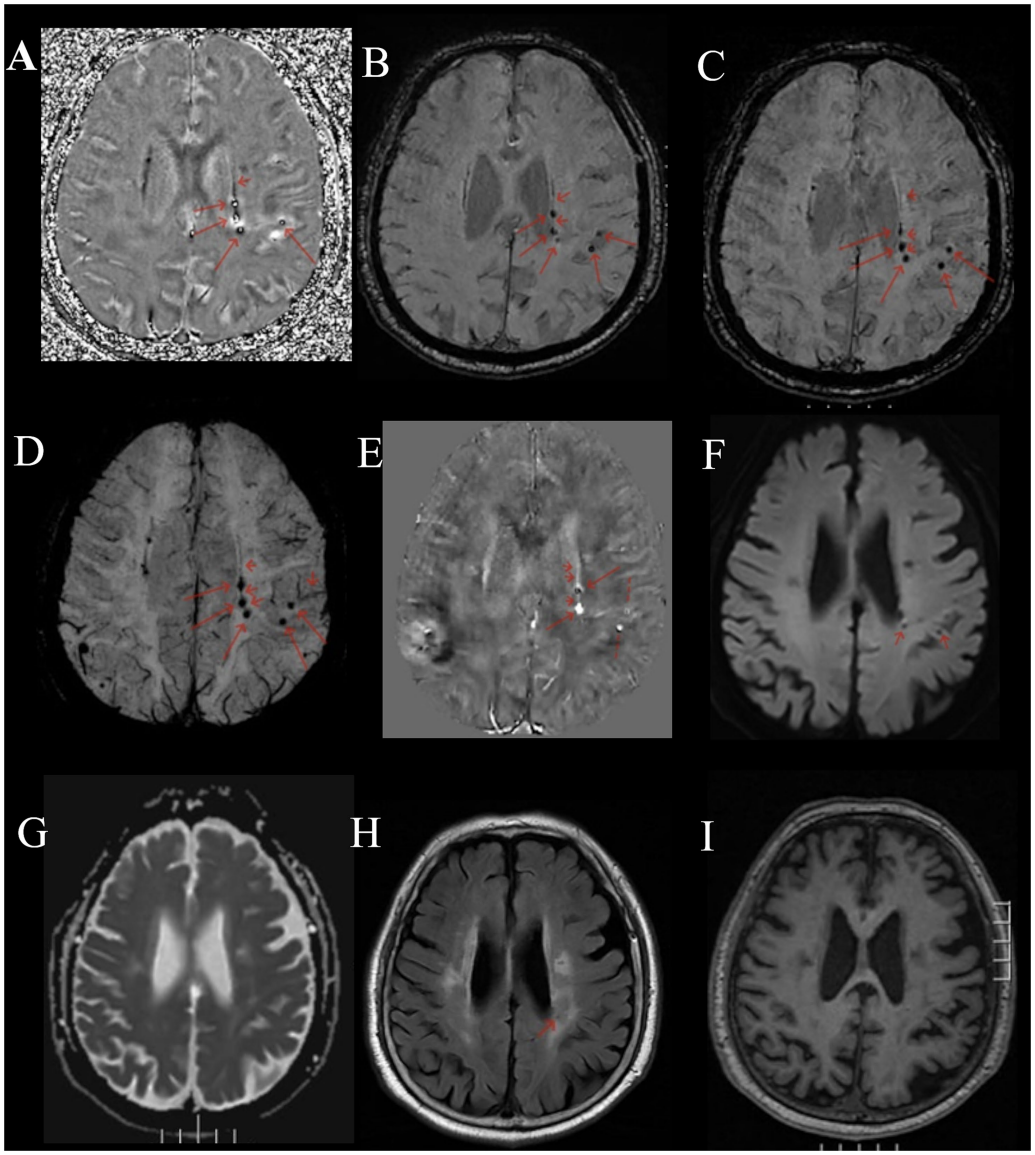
Male, 73 years old, with right limb motor impairment for 15 years and dizziness for 1 day. (A) Phase map. (B) Amplitude map. (C) SWI image. (D) MinIP image with TE of 22.5 ms. (E) QSM image. (F) DWI image. (G) ADC map. (H) FLAIR image. (I) T1WI image. (A–D) Round-like hypointense signals can be seen in the left periventricular region and the left parietal cortex, with hyperintense signals in the center, forming a typical “target sign” (long red arrow). The surrounding lesions show hypointense signals from the drainage of the left subependymal vein and parietal cortical vein (short red arrow). (E) QSM shows two round-like abnormal signals in the left periventricular region. The central part is the hyperintense central vein, the middle is hypointense, and the outermost edge is slightly hyperintense or hyperintense, forming the most typical “target sign” (long red arrow). The surrounding lesions have strip-like hyperintense subependymal vein drainage (short red arrow) passing through two BCTs (long red arrow). The left parietal cortex shows round-like lesions with hypointense in the center and hyperintense at the edge, forming a typical “target sign” (dashed long line). (F) DWI image, showing punctate hypointense signals in the left periventricular region and parietal cortex (short red arrow). (G) Shows slightly hyperintense signals. (H) Shows hypointense signals (short red arrow). (I) T1WI image, showing slightly hypointense signals.
